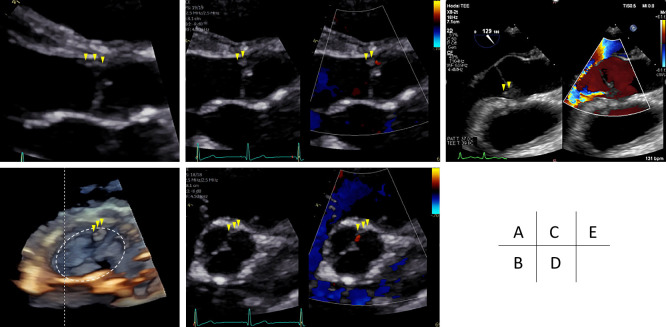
Transthoracic and transesophageal echocardiographic findings. (A) Long-axis (LAX) view of the aortic valve (AoV) on TTE showing a 4.4×1.0-mm thread-like structure attached to the line of valve closure (yellow arrow heads). The structure appears to go back and forth between the left ventricular (LV) side and the aortic side. (B) Three-dimensional echocardiographic view from the LV side showing a Lambl’s excrescence (LE) (TTE). The LE arises from the commissure between the right coronary cusp (RCC) and left coronary cusp (LCC), projecting toward the LV side after AoV closure (yellow arrow heads). (C) LAX view and (D) short-axis view of the AoV on transthoracic echocardiography (TTE), with color Doppler imaging showing mild aortic regurgitation (AR) originating near the commissure of the RCC and the LCC. A structure adheres to the same site (yellow arrow heads). (E) LAX of the AoV on transesophageal echocardiography (TEE) (mirror image of panel C).
